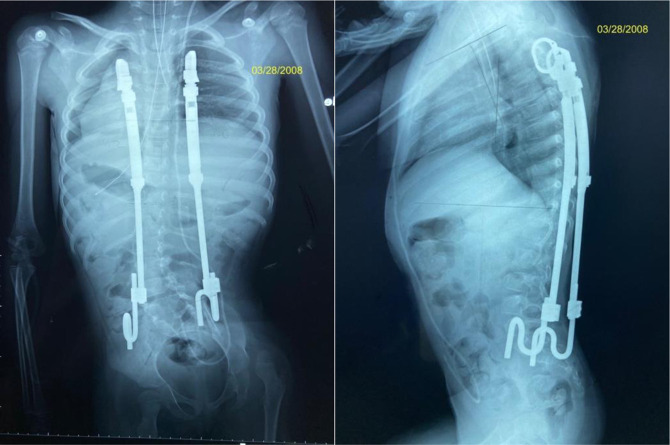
Radiography showing the status post bilateral rib-to-pelvis growing system.
